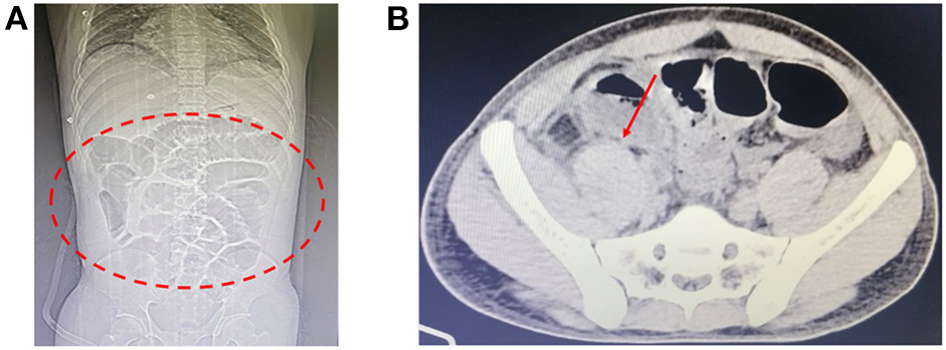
Computed tomography scans. (A) Coronal view showing dilated intestinal loops (red dashed circle). (B) Axial view indicating the presence of intraluminal fluid (red arrow).
